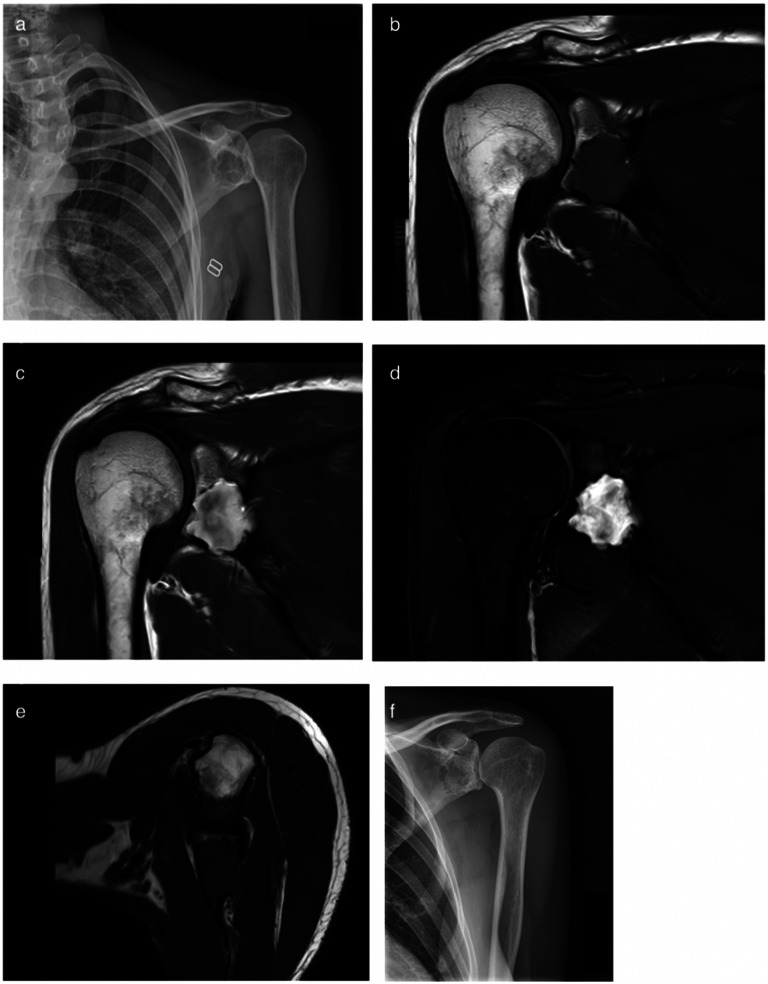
(a) X-ray shows osteolytic lesion in the glenoid with sclerotic border and trabeculation. (b and c) Frontal images in T1WI show an intraosseous lesion with clear cortical breach inferiorly and discrete soft tissue expansion outside the glenoid. There is contrast enhancement after administration of gadolinium. (d) There is hyperintense signal in T2WI. (e) Axial images also show an isointense signal to muscle tissue and cortical breach laterally. (f) No recurrence is seen on X-ray after 12 months.
